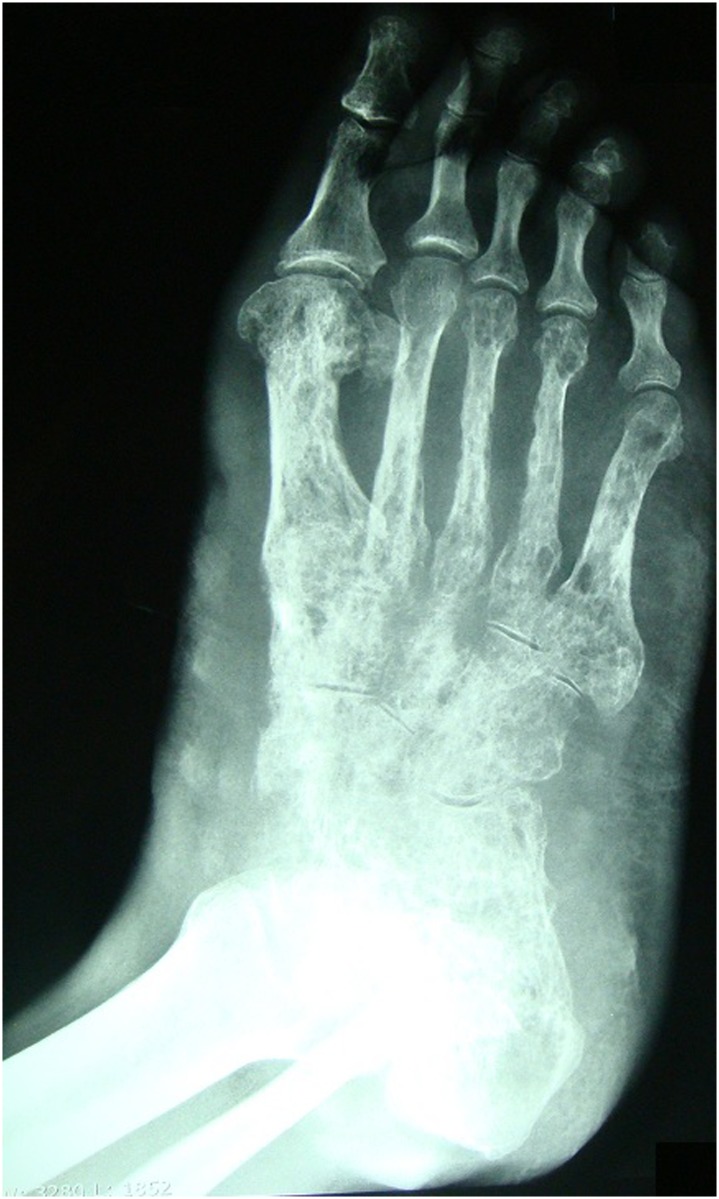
Radiography showing bone destruction.
